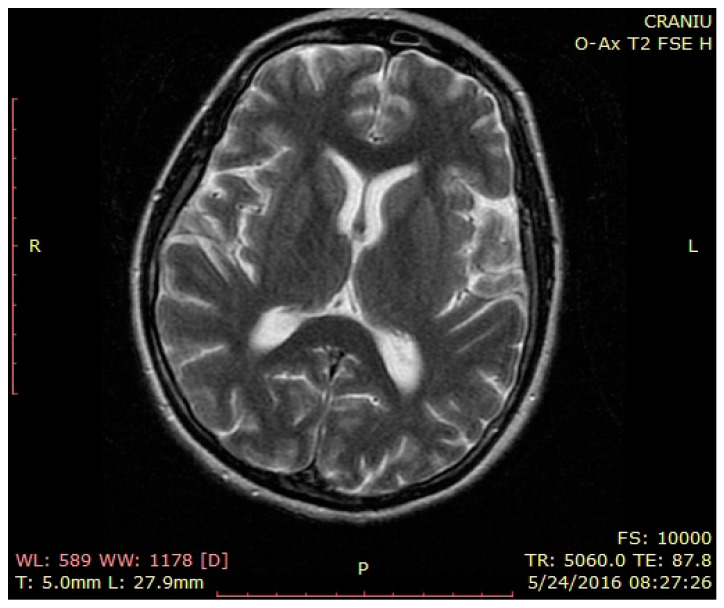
Brain MRI—normal findings (T2 weighted brain scan), axial insular image 2.
